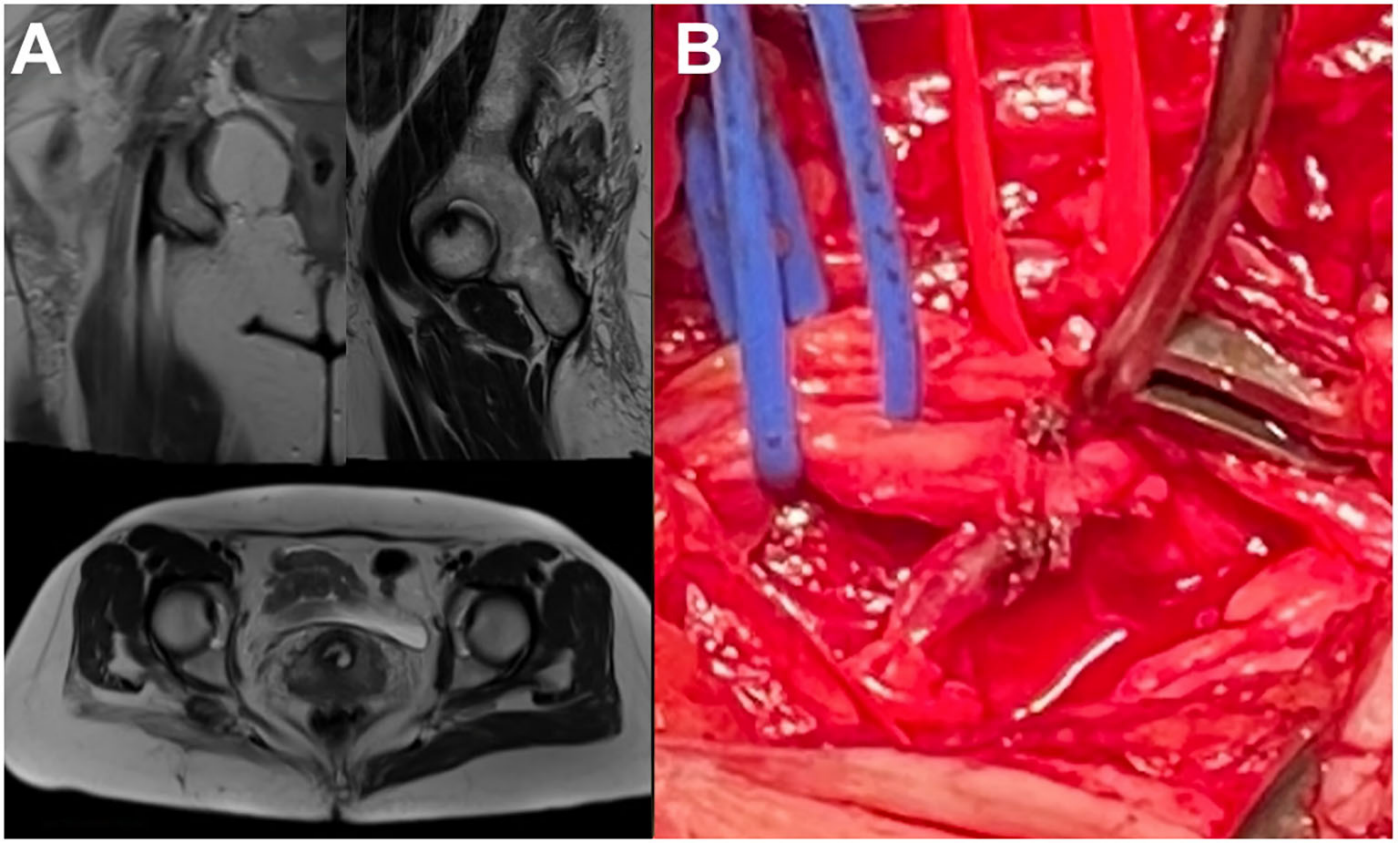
Isolated sciatic nerve endometriosis. (A) MRI finding. (B) Intraoperative finding (transgluteal approach).
